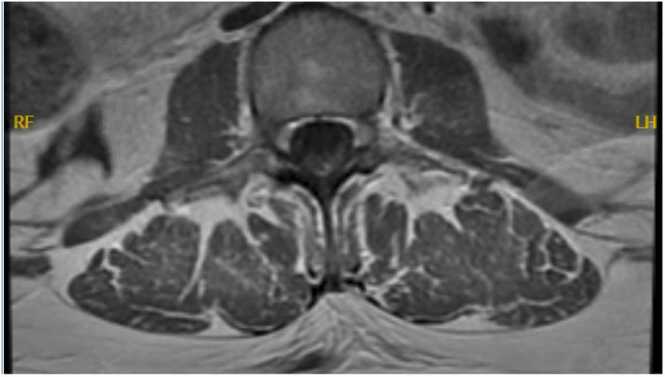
Repeat MRI whole spine w/wo contrast demonstrating a linear enhancement in the dorsal aspect of the thecal sac from L2 to L4.
